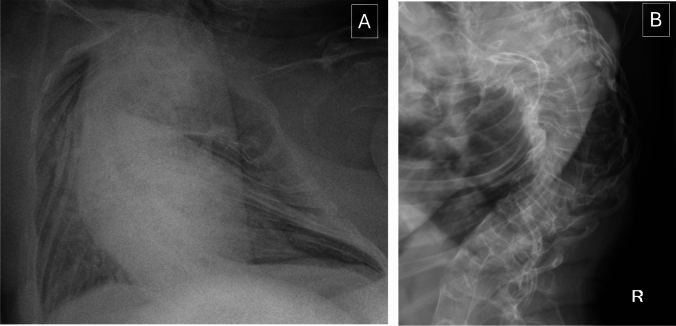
Radiographs of spine and chest. Panel A shows a chest X-ray prior to surgery demonstrating the pre-existing chest deformity. Panel B shows a lateral X-ray of the thoracic and upper lumbar spine demonstrating kyphoscoliosis
